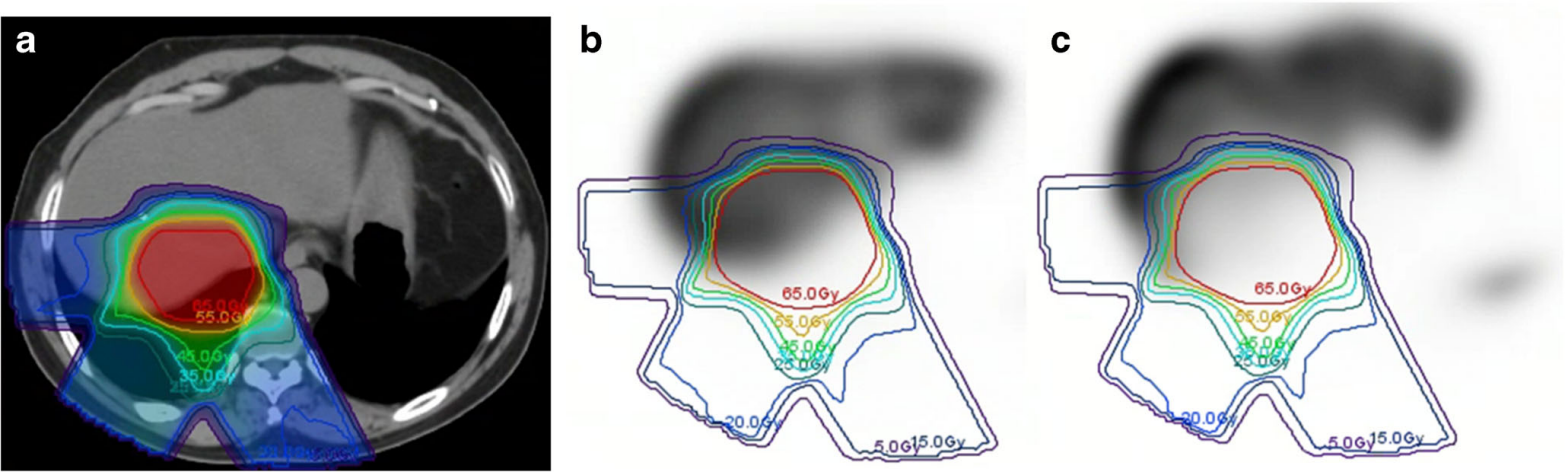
Planning CT (a) and longitudinal ^99m^Tc-sulfur colloid SPECT images, baseline (b) and 1 month post-treatment (c), acquired for a patient who received proton therapy for liver cancer. This image was reproduced from Price et al. with permission [70]
